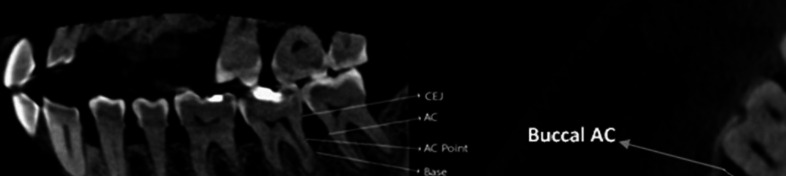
CBCT showing the radiographic measurement of the bone defect: (A) Sagittal view of CBCT showing the reference points; CEJ, base of the defect, alveolar crest (AC), and AC point.(B) Axial view of CBCT showing the BL width of the defect was measured in the axial plane as the horizontal distance between the most coronal point for the buccal and lingual ACs
